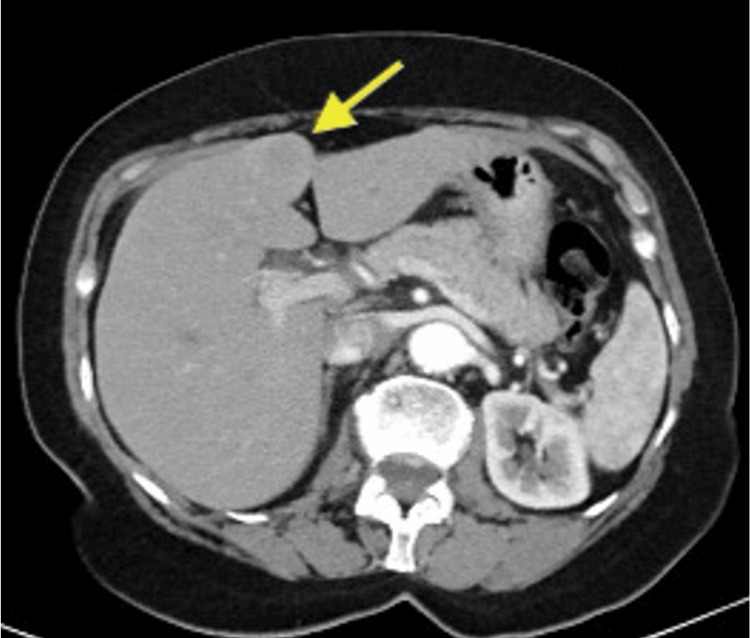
Contrast-enhanced computed tomography (CT), axial view, demonstrating a well-circumscribed 2.5-cm solid lesion in hepatic segment IVb (yellow arrow) with peripheral enhancement.
